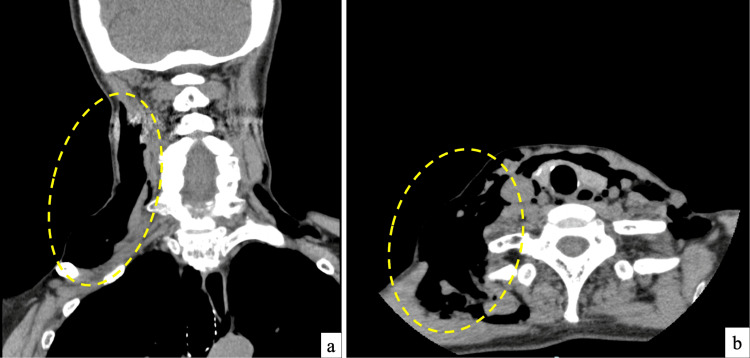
(a, b) Postoperative CT images demonstrating the presence of free air in the right cervical region CT: computed tomography
